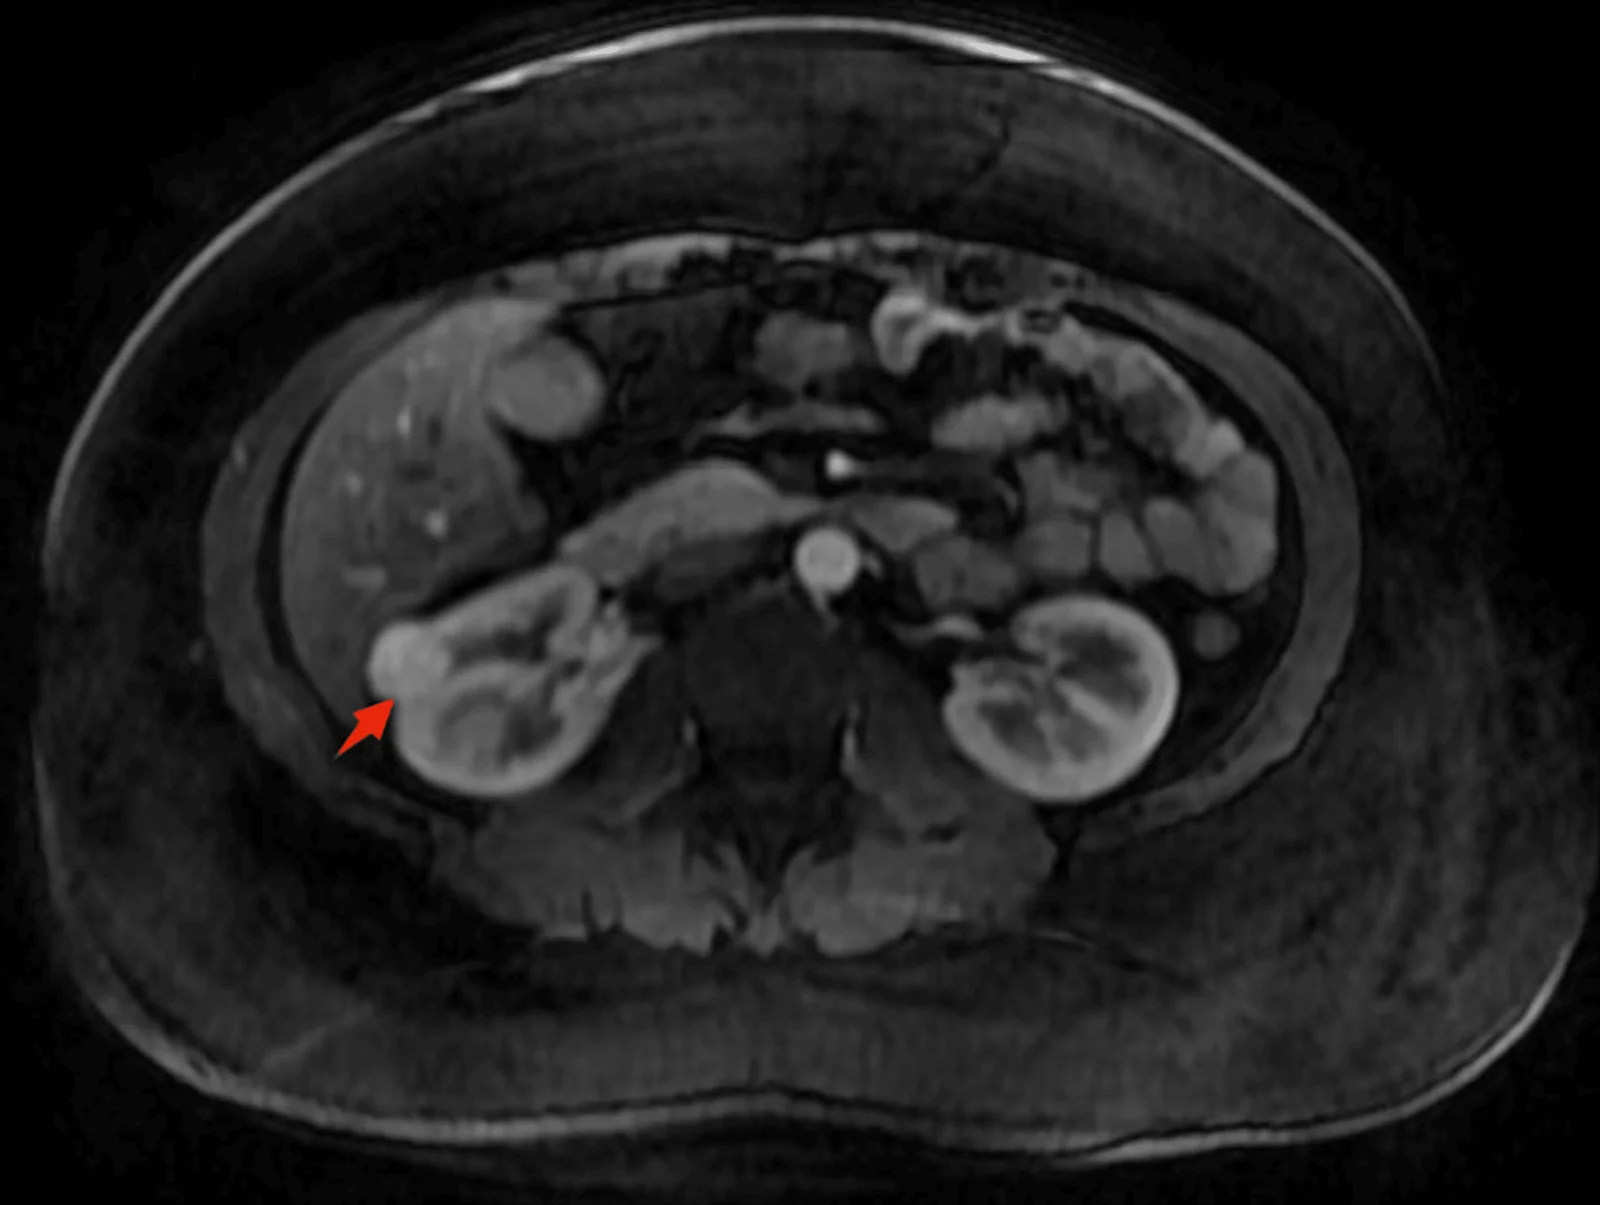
Arterial phase of contrast-enhanced MRI The arrow indicates a lesion with significant and uniform enhancement, suggesting a high blood supply MRI: magnetic resonance imaging
